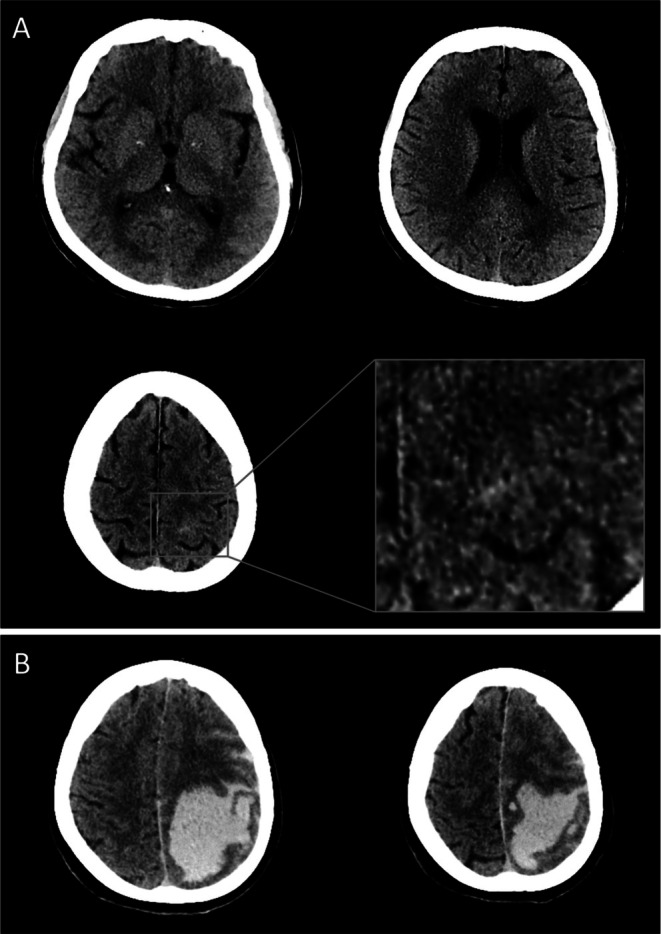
Radiological presentation. Initial cranial CT scan demonstrates moderate periventricular and deep white matter lucencies indicative of small vessel disease (A), with a tiny sulcal hyperdensity noted during retrospective evaluation (inlet of A). Urgent follow‐up CT scan after IVT demonstrates a large frontal–parietal ICH with subarachnoid extensions and finger‐like projections (B).
